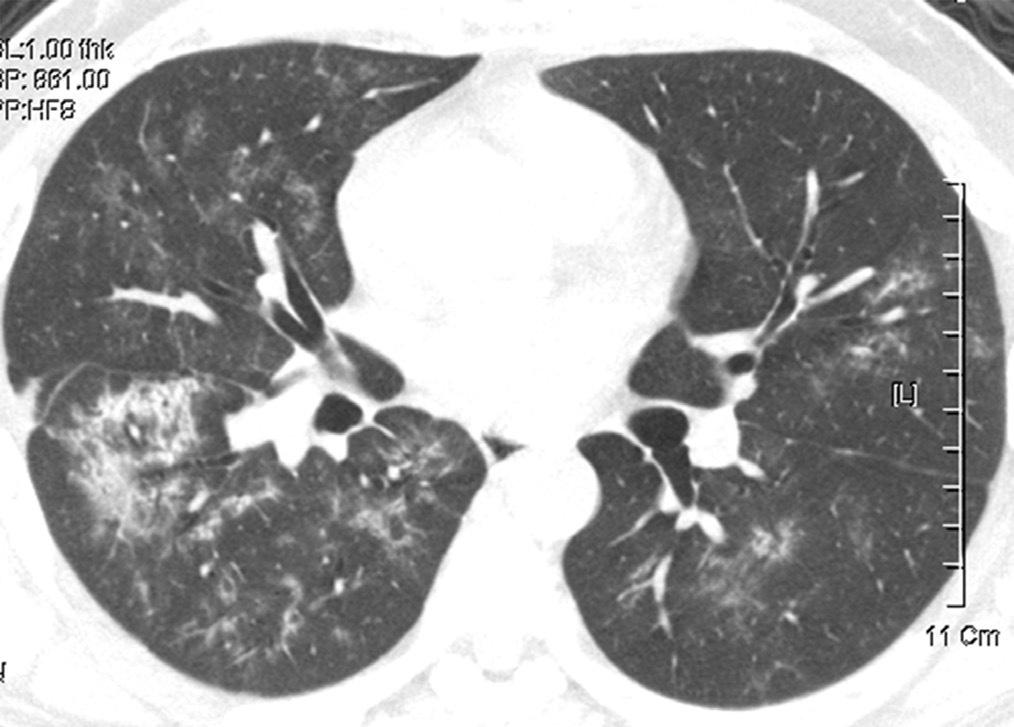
A 43-year-old male with SARS-CoV infection. Chest CT obtained 25 days after symptom onset shows bilateral diffuse ill-defined centrilobular and ground-glass opacity nodules in both lungs. Also, note focal consolidation in right lower lobe.
